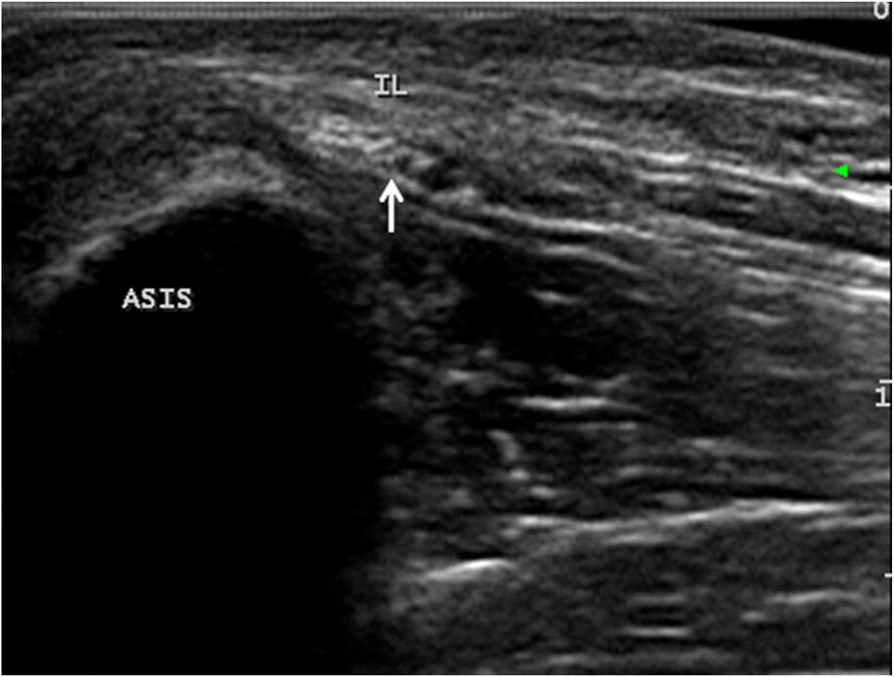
Transverse ultrasound image shows the nerve passing under the inguinal ligament. ASIS: anterior superior iliac spine; LFCN: lateral femoral cutaneous nerve; IL: inguinal ligament.
